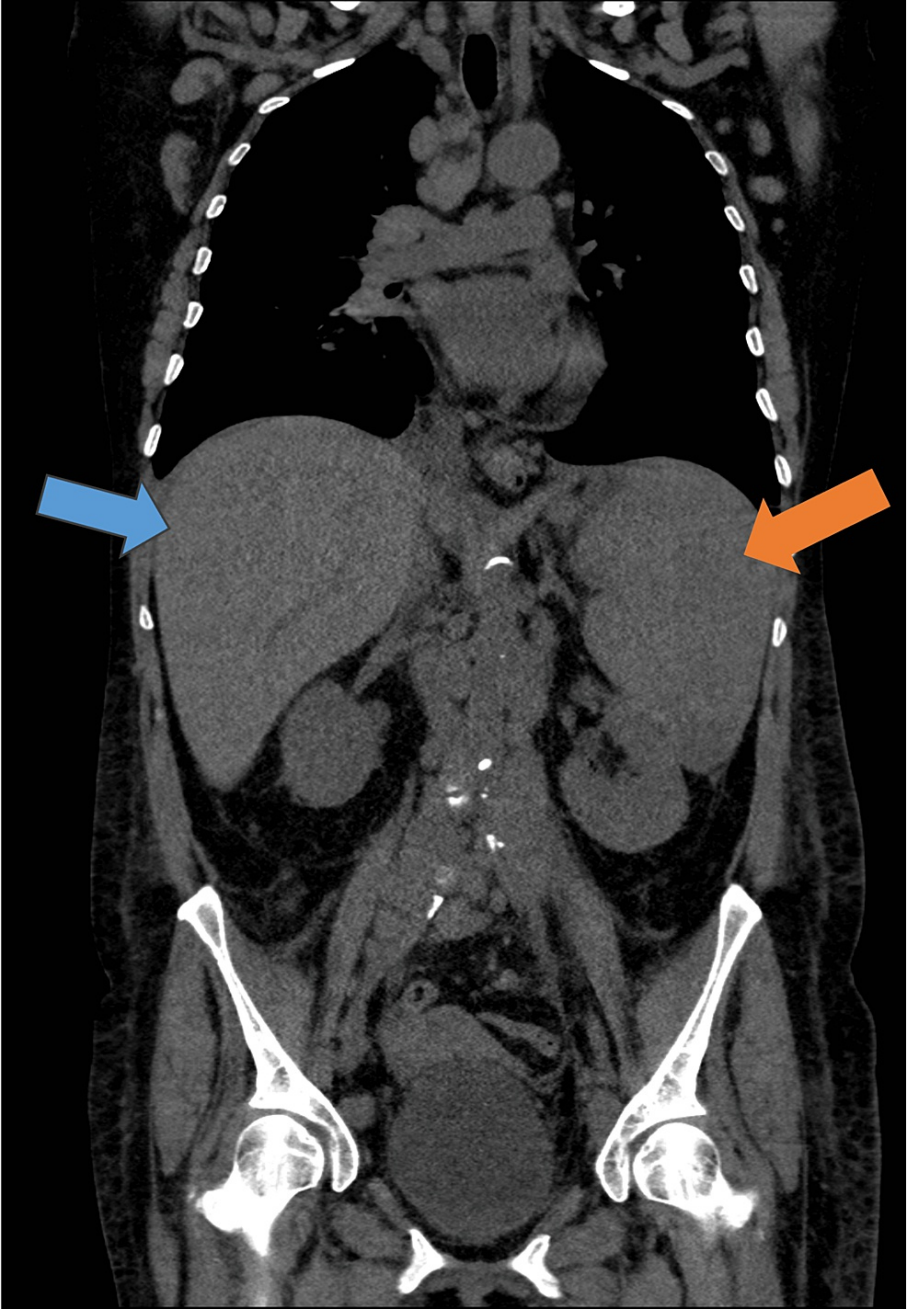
Computed tomography Blue arrow, hepatomegaly; orange arrow, splenomegaly
